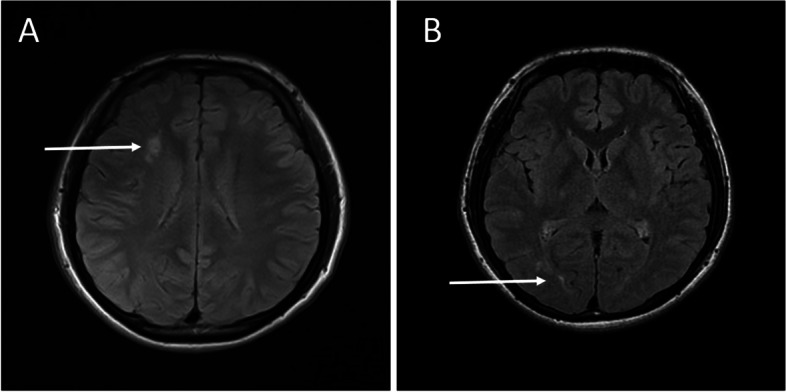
T2 brain MRI revealed mild, asymmetrical, non-enhancing white matter lesions, mainly in the right frontal (A) and posterior (B) periventricular regions
